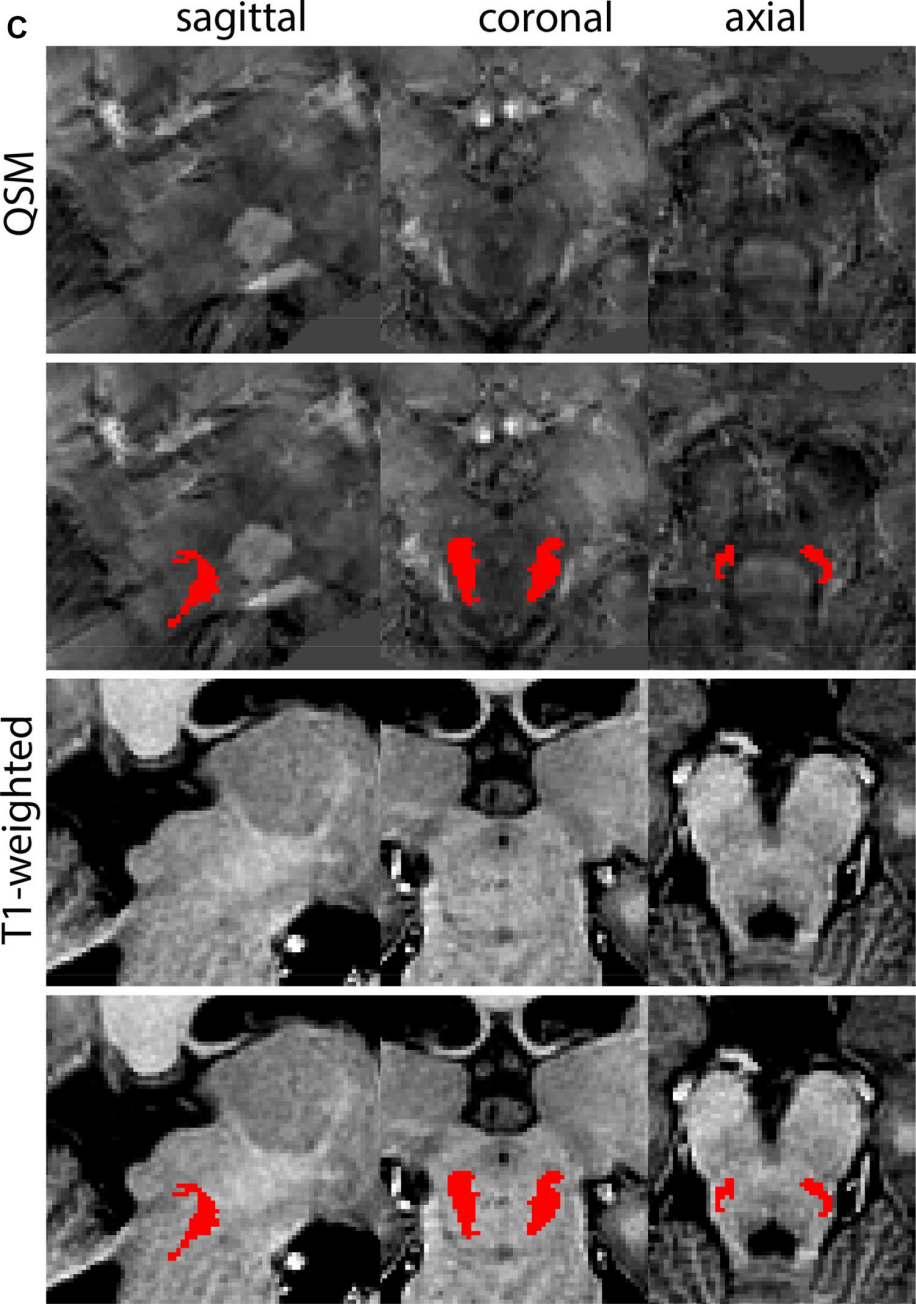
a The pedunculopontine nucleus (PPN) is a grey matter structure located in a white matter rich area. As a result it presents as a relatively hypointense structure on T1-weighted images. b The pedunculopontine nucleus (PPN) is a grey matter structure located in a white matter rich area. As a result it presents as a relatively hypointense structure on T1-weighted images. Note the appearance of the PPN in the sagittal plane at this level. c The pedunculopontine nucleus is a grey matter structure located in a white matter rich area. As a result it presents as a relatively hypointense structure on T1-weighted images. Note the difference in the sagittal appearance with (b)
